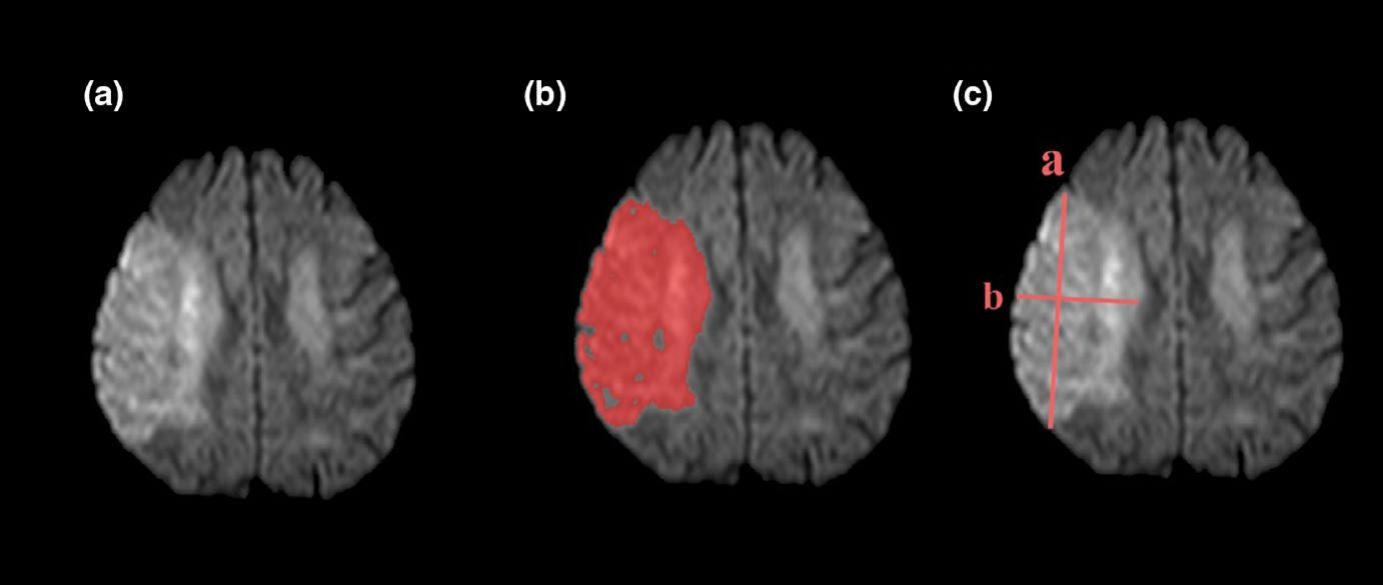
(A) Example patient with a right‐sided middle cerebral artery (MCA) stroke visible on diffusion‐weighted imaging (DWI) at baseline. (B) Based on manual delineation of the acute lesion, lesion volume was estimated to be 154.9 ml. (C) The largest diameter (*a* = 9.2 cm) and the corresponding largest orthogonal diameter (*b* = 4.8 cm) resulted in an od‐value of 44.2 (i.e., correct estimation of lesion size > 100 ml). Calculation of ABC/2 = (9.2 × 4.8) × 13 slices × 0.25 cm slice thickness resulted in an estimated volume of 143.5 ml (i.e., correct estimation of lesion size > 100 ml)
